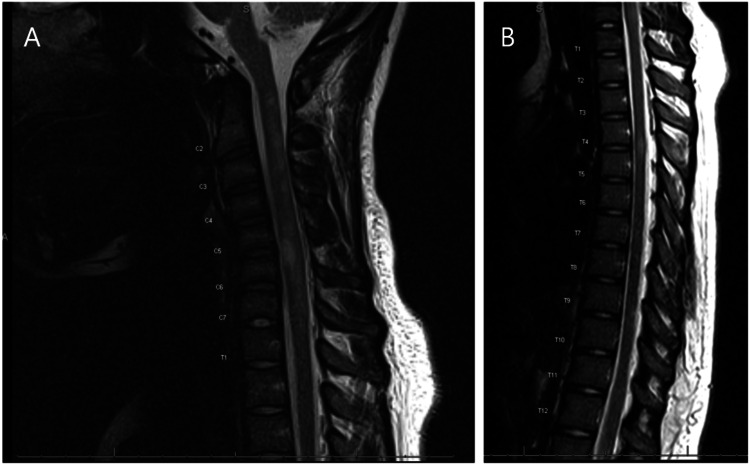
Spine MRI demonstrating multifocal short-segment cervical and thoracic spinal cord demyelinating lesions. Spine MRI. (A) Sagittal T2-weighted cervical spine image demonstrating multifocal short-segment intramedullary T2 hyperintense lesions with an active enhancing lesion reported at C5–C6. (B) Sagittal T2-weighted thoracic spine image demonstrating a short-segment T2 hyperintense cord lesion at T4–T5 without enhancement.
